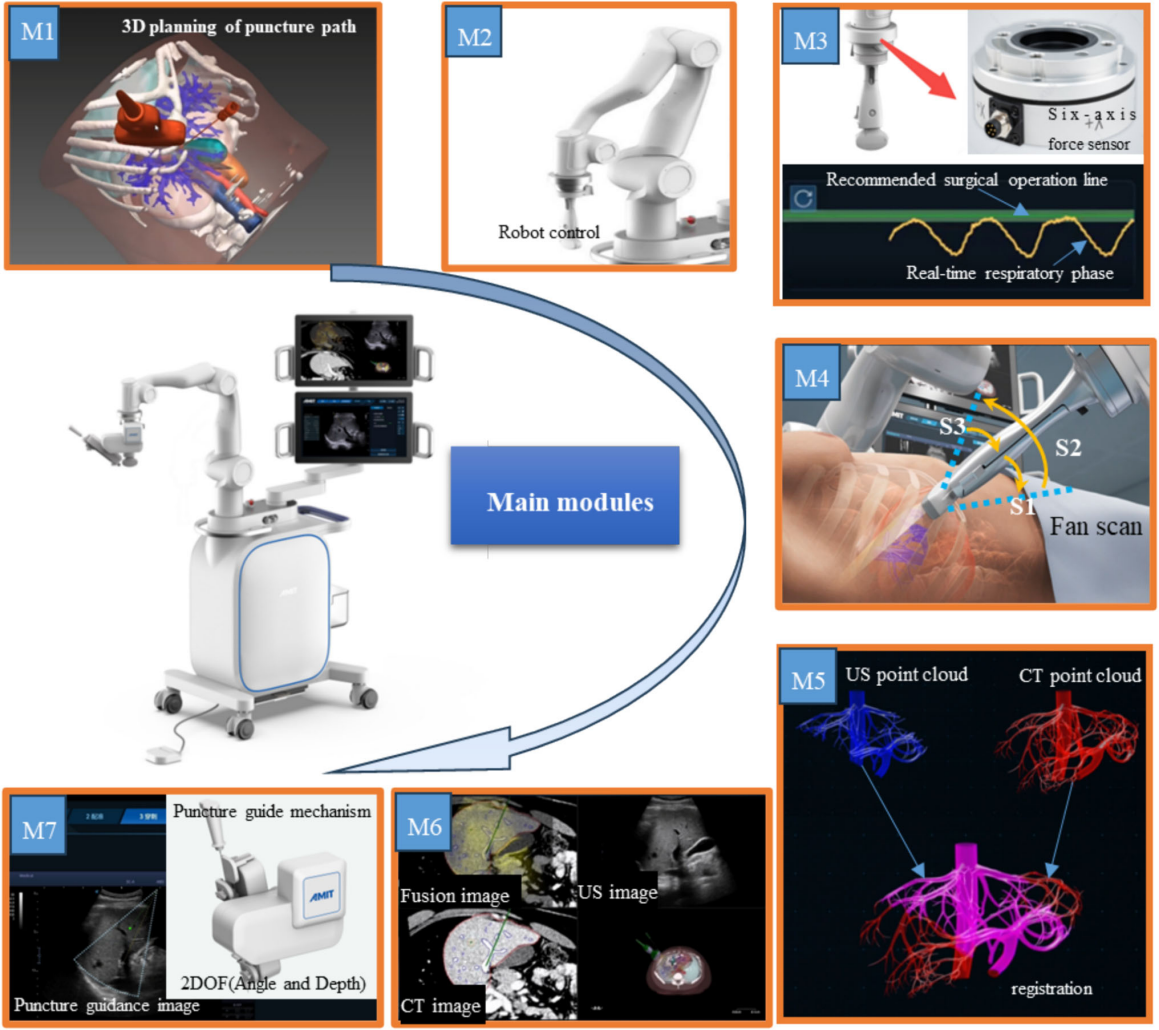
Main modules of the robot. First, the robot plans the puncture surgery before the operation to assist doctors (M1). Second, the doctor can switch between free dragging mode and automatic motion control of the surgical robot (M2). The robot has seven degrees of freedom, and its end is rigidly connected to the US probe. The system can use the positional data of the robotic arm and the information of US images in place of data from the external optical sensors of traditional surgical robots for tumor localization. Third, the force exerted on the ultrasound probe is acquired through a six-axis force sensor integrated with the robot arm (M3). Based on the acquired force data, the respiratory phase of the patient is identified, facilitating the acquisition of US images during the inspiratory phase, and enabling precise navigation of the puncture trajectory. Fourth, the system reconstructs the 3D image using the US probe (M4). The system directs the US probe to perform a fan scan, capturing US information of the liver tissue along the path of the probe. The reconstruction algorithm uses the obtained US images and the position data of the robotic arm to construct a 3D US model. This model is then used to convert real-time US blood vessels into a point cloud. The blood vessel is estimated and converted from the shape to point cloud using the previous AI model [15] which is constructed by the U-Net model and learnt the labeled US image by surgeons. Fifth and sixth, the system registers the image (M5) and fuses 3D US and CT images using this paper’s following method (M6). Finally, the robot performs puncture surgery (M7)
